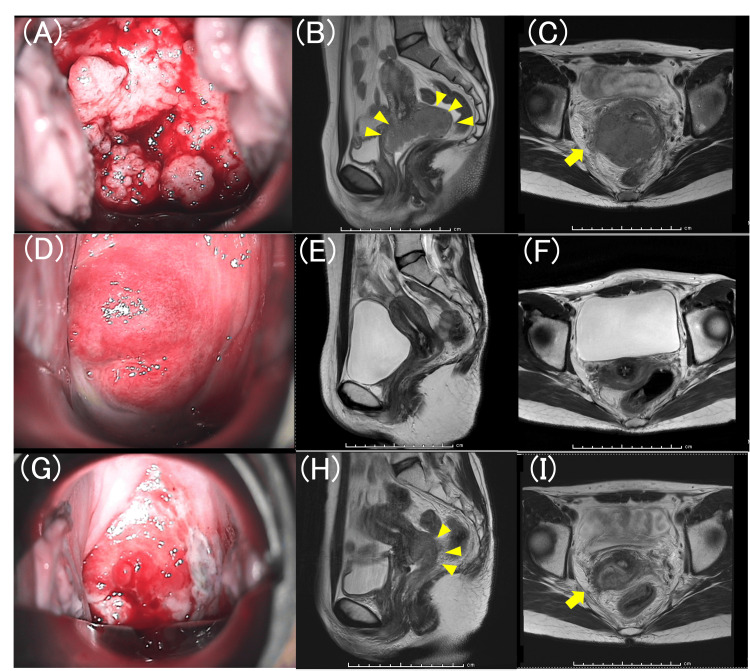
Colposcopic and MRI findings at initial presentation after CCRT and at recurrence. (A) Colposcopy at initial presentation; (B) Sagittal T2-weighted MRI at initial presentation; (C) Axial T2-weighted MRI at initial presentation; (D) Colposcopy at completion of CCRT; (E) Sagittal T2-weighted MRI at completion of CCRT; (F) Axial T2-weighted MRI at completion of CCRT; (G) Colposcopy at recurrence; (H) Sagittal T2-weighted MRI at recurrence; (I) Axial T2-weighted MRI at recurrence. The primary cervical tumor is indicated by arrowheads, and right parametrial invasion is indicated by arrows. MRI: magnetic resonance imaging; CCRT: concurrent chemoradiotherapy
